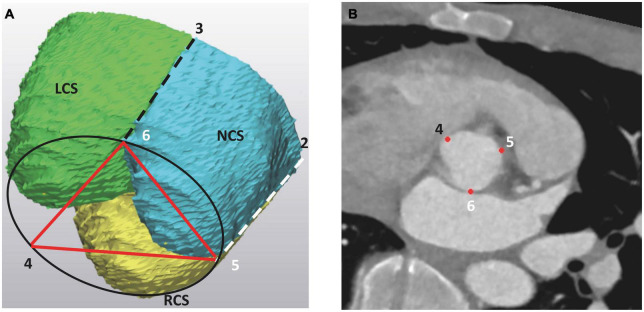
The basal landmarks of the aortic root at the level of the aortic root base (AoB), as seen (A) on the 3D model of the aortic root and (B) on the angio CT scan. The basal landmarks “*456*” correspond to the deepest points at each IVT and are vertical projections of the three commissures into the AoB. On the 3D model, the LCS is marked as green, the RCS is yellow and the NCS is blue. The projection of the left and right commissure to the aortic root base is indicated by dashed lines. LCS, left coronary sinus; RCS, right coronary sinus; NCS, non-coronary sinus.
